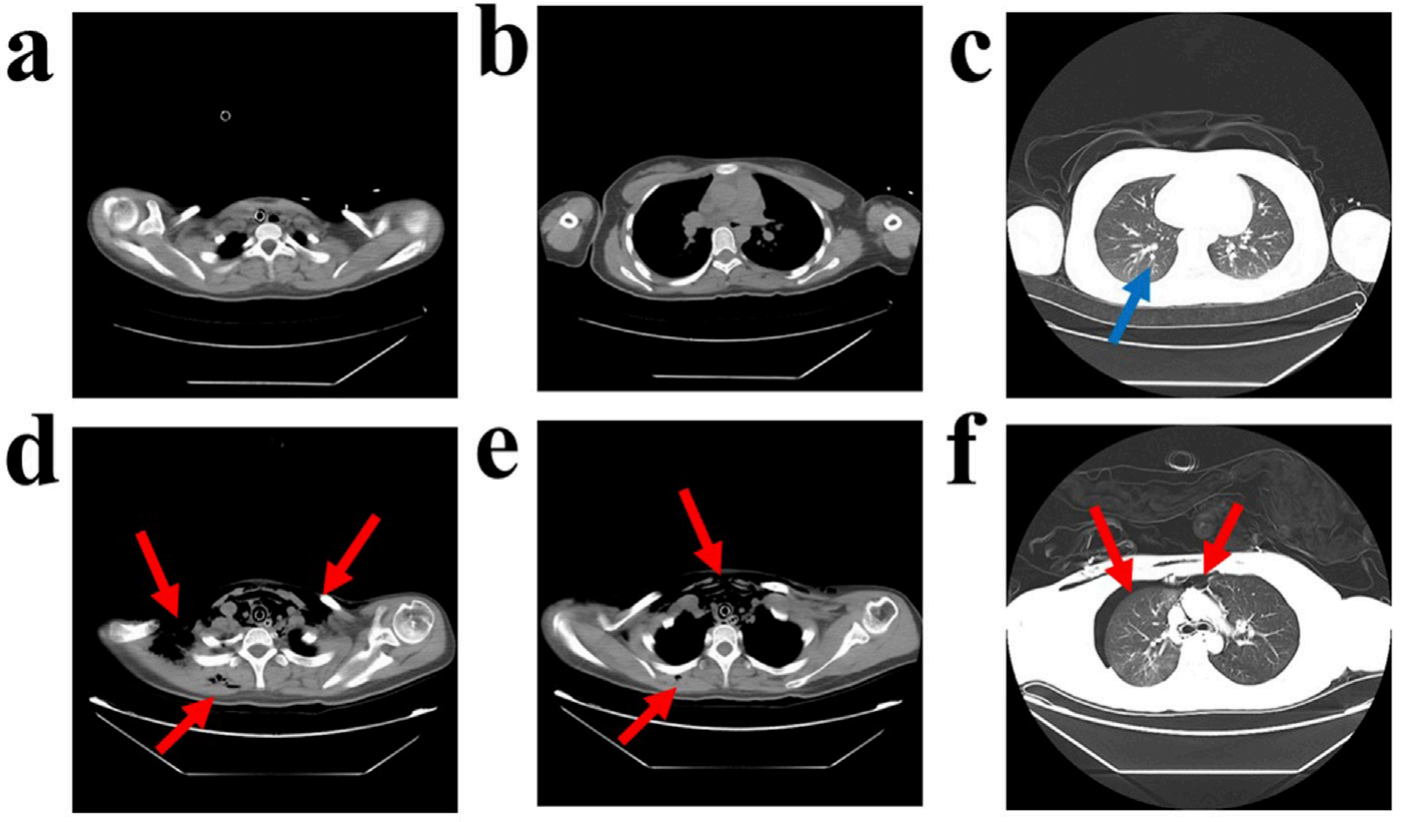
Computed Tomography Scan of the Patient’s Chest. A computed tomography (CT) scan of the chest taken on the first day of admission revealed minor inflammation in the lower lobe of the left lung, as indicated by the blue arrows (a–c). The same CT scan also showed diffuse air accumulation in the bilateral neck, shoulders, anterior chest wall, thoracic cavity, mediastinum, and subcutaneous air in the right dorsum, with approximately 20% compression of the right lung. Additionally, multiple patchy ground-glass opacity areas were observed in the right upper lobe and the lower lobes of both lungs, with indistinct borders, as indicated by the red arrows (d–f).
